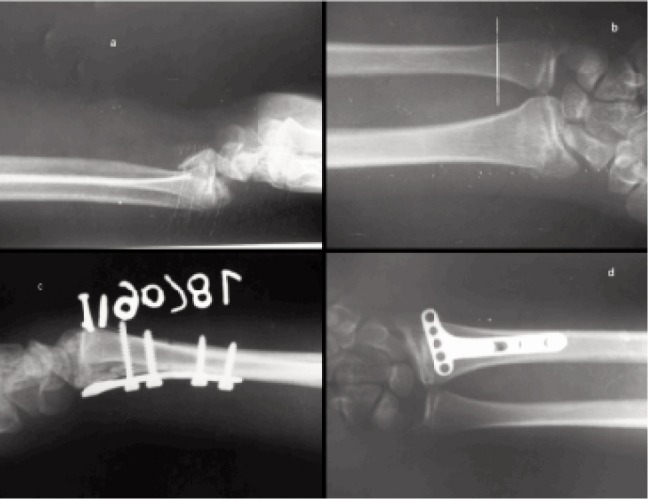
Radiographs a) Lateral view: intra articular distal end radius fracture, b) Antero posterior view: intra articular distal end radius fracture, c) immediate postoperative lateral view showing well reduced intra articular fragment, d) postoperative AP view showing cortical screws only proximal to fracture site, with empty distal screw holes.
